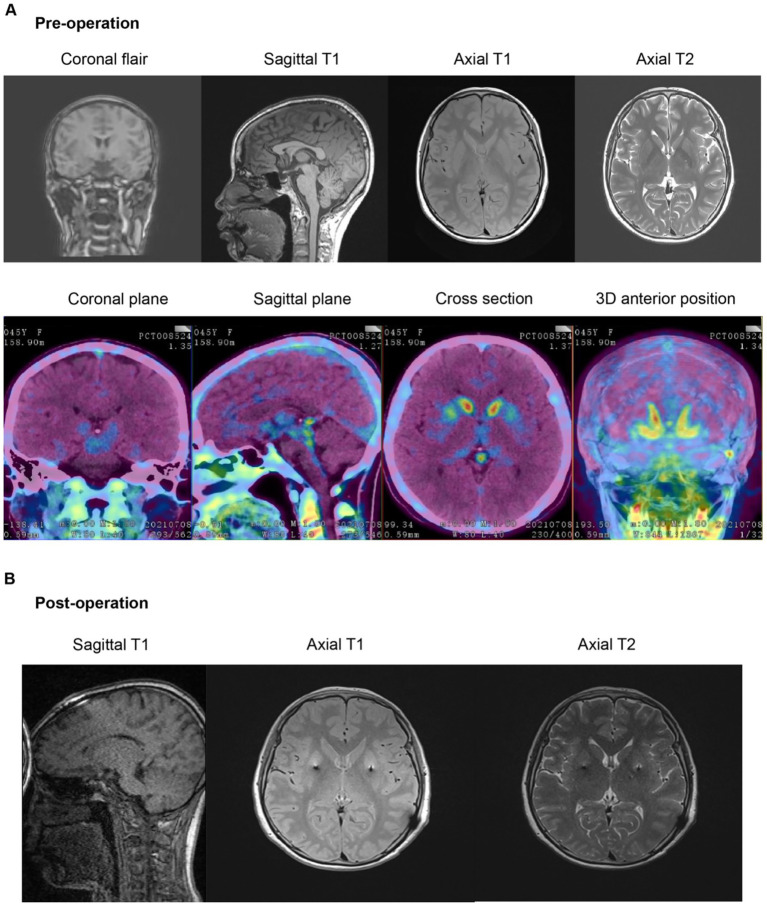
Preoperative and postoperative neuroimaging results in Case 1. (A) Preoperative brain magnetic resonance and PET/CT neuroimaging results. PET/CT examination showed that 18F-DOPA uptake was significantly reduced in the bilateral substantia nigra, caudate nucleus, and lentiform nucleus, especially in the bilateral lentiform nucleus. (B) MRI on post-operation. Axial T1 and T2 Flair-weighted MRI sequences showing postoperative images of the brain parenchyma and basal ganglia.
